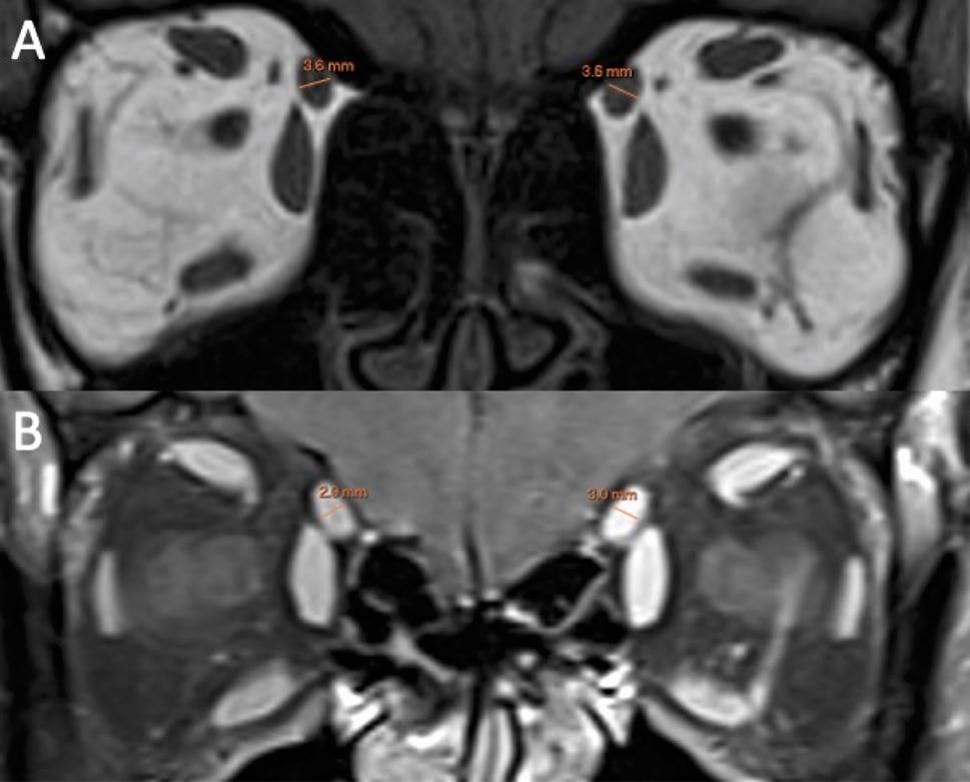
Coronal T1-weighted MRI (A) and fat suppressed contrast-enhanced T1-weighted MRI (B) showing the measurements of the superior oblique muscle
